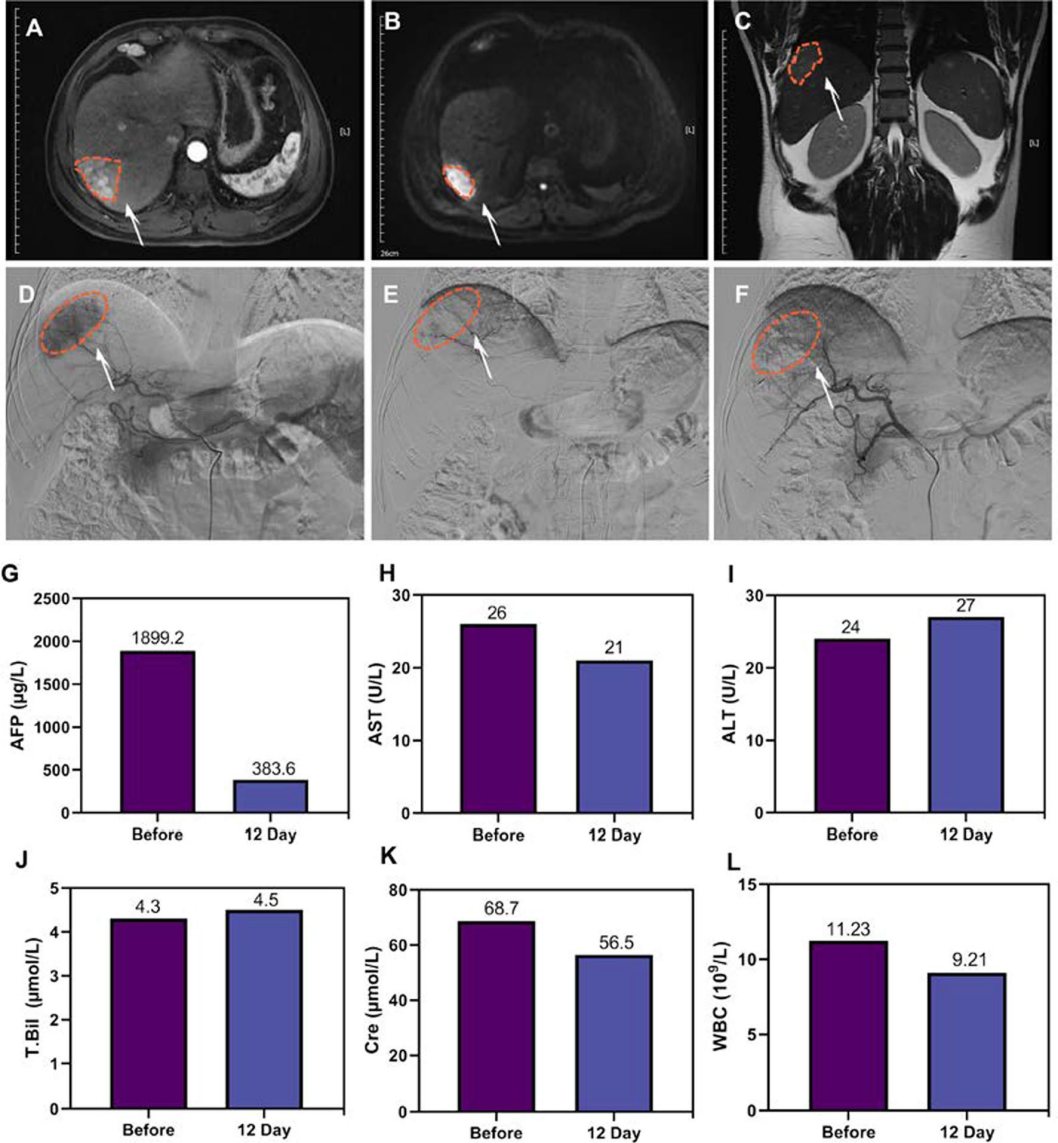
Embolism and safety evaluation in clinical case of HCC. A–C MRI showed that a liver tumor at the right posterior lobe with a maximum diameter of 4.3 cm was observed (arrow): 4 × 2.4 × 4.3 cm^3^ (A: arterial phase, B: venous phase, C: coronal). D–F DSA angiography and SHIFT&ICG embolization (D: before embolization, E: during embolization, F: after embolization). G Blood AFP value (reference interval: 0.0 ~ 20.0 µg/L) before and 12 days after TAE. H–L Blood AST (reference interval: 15.0 ~ 40.0 U/L), ALT (reference interval: 9.0 ~ 50.0 U/L), T. Bil (reference interval: 1.7 ~ 26.0 µmol/L), Cre (reference interval: 57.0 ~ 97.0 µmol/L) and WBC value (reference interval: 3.5 ~ 9.5 10E9/L) before and 12 days after TAE
